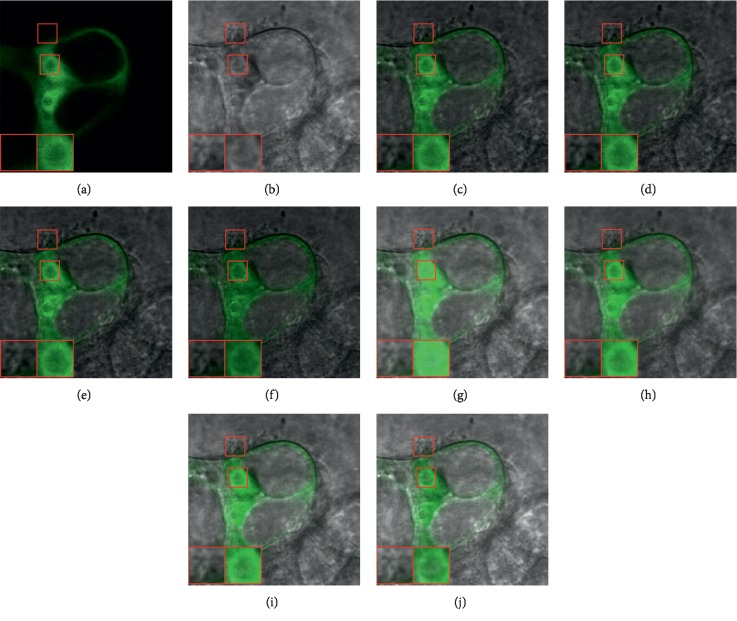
The second set of GFP and phase-contrast image fusion results. (a) GFP image. (b) Phase-contrast image. (c) DTCWT. (d) CVT. (e) NSCT. (f) SR. (g) CNN. (h) SFL-CT. (i) CST. (j) Proposed.
